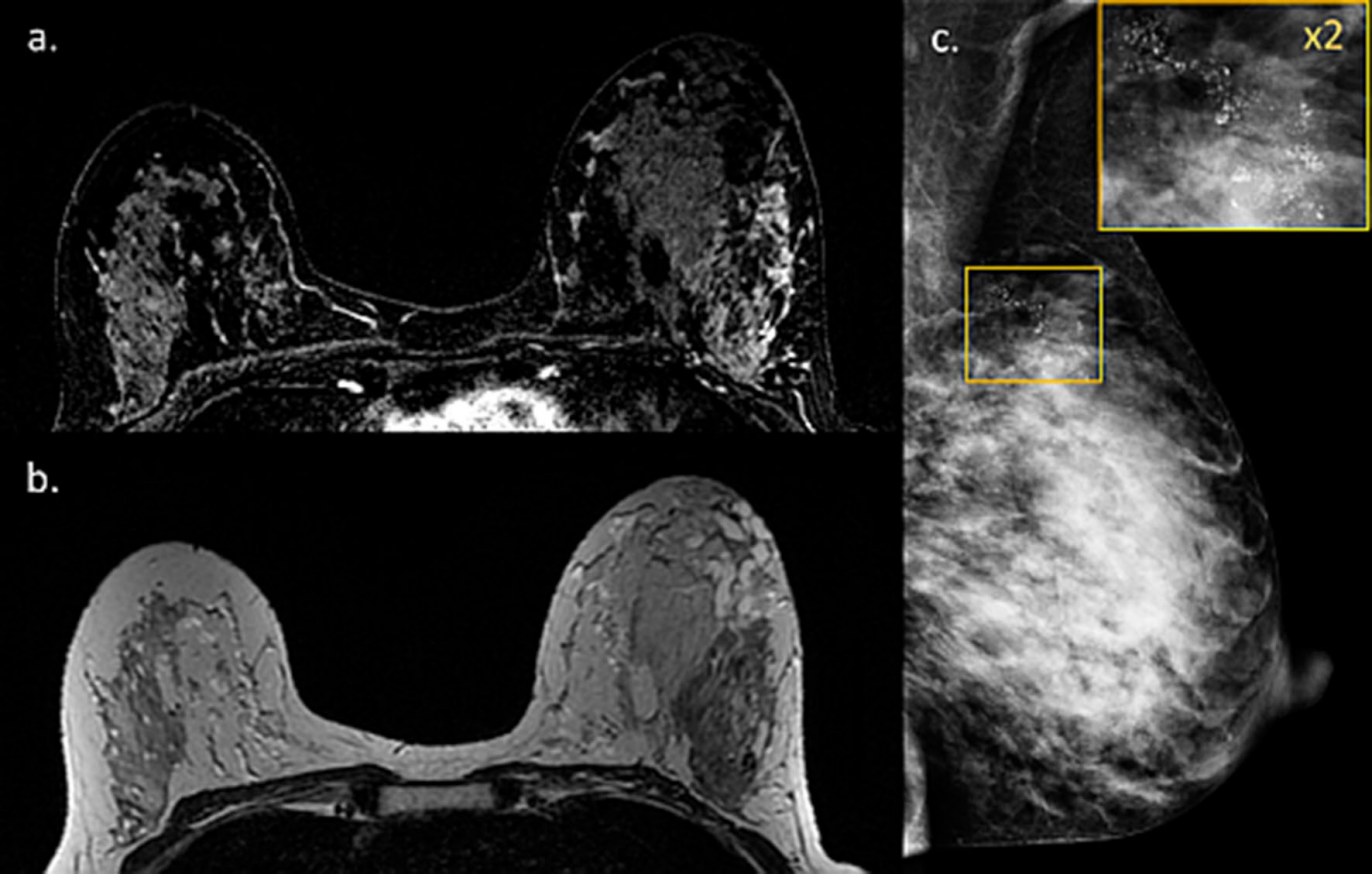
MRI of DCIS during lactation. Images of a 40-year-old PABC patient (lactating for 4 months) with DCIS confirmed on operation are presented. The patient underwent screening mammography with adjuvant breast US (not presented) due to the extremely dense breasts on mammography (BI-RADS D) (c). Mammography revealed suspicious pleomorphic micro-calcifications in segmental distribution along 6 cm in the left upper outer quadrant (c). Pre-operative MRI showed NME on top of the surrounding prominent lactation-induced BPE, typical for DCIS, in agreement with the calcifications location (a). Furthermore, an excellent tumor delineation was afforded by non-fat surpassed T2-weighted image, showing hypo-intense region in the tumor area (b)
